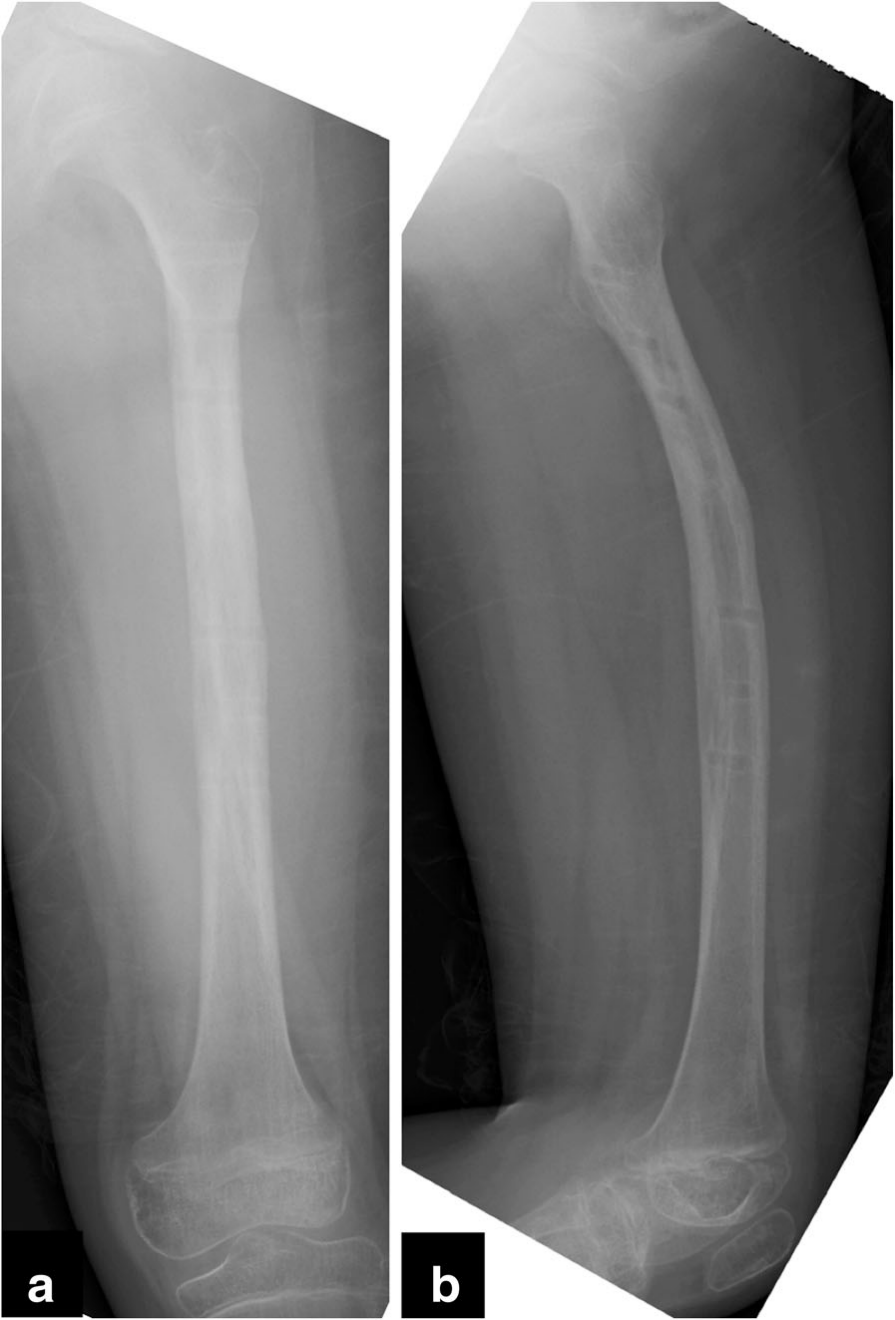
Radiographs of the left femur at the 12-week follow-up after the first operation. a the anteroposterior view, b the lateral view
